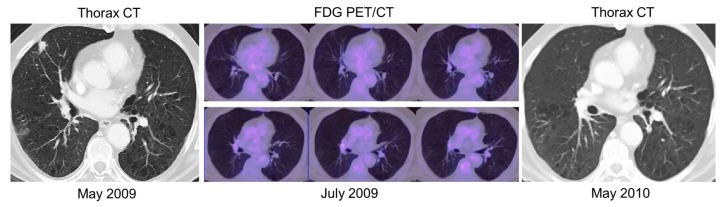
A 74 year-old man with a history of bladder cancer underwent thorax and abdominal contrast-enhanced CT (ceCT) scan, which showed suspected lung recurrence. Later, positron emission tomography/computed tomography (PET/CT) was negative for any recurrence, particularly in the lung. A further thorax-abdominal ceCT scan, performed after 1 year later, revealed the disappearance of the lung nodule. Therefore, PET/CT was able to change therapeutic management, from a potential curative intent to an observational strategy.
